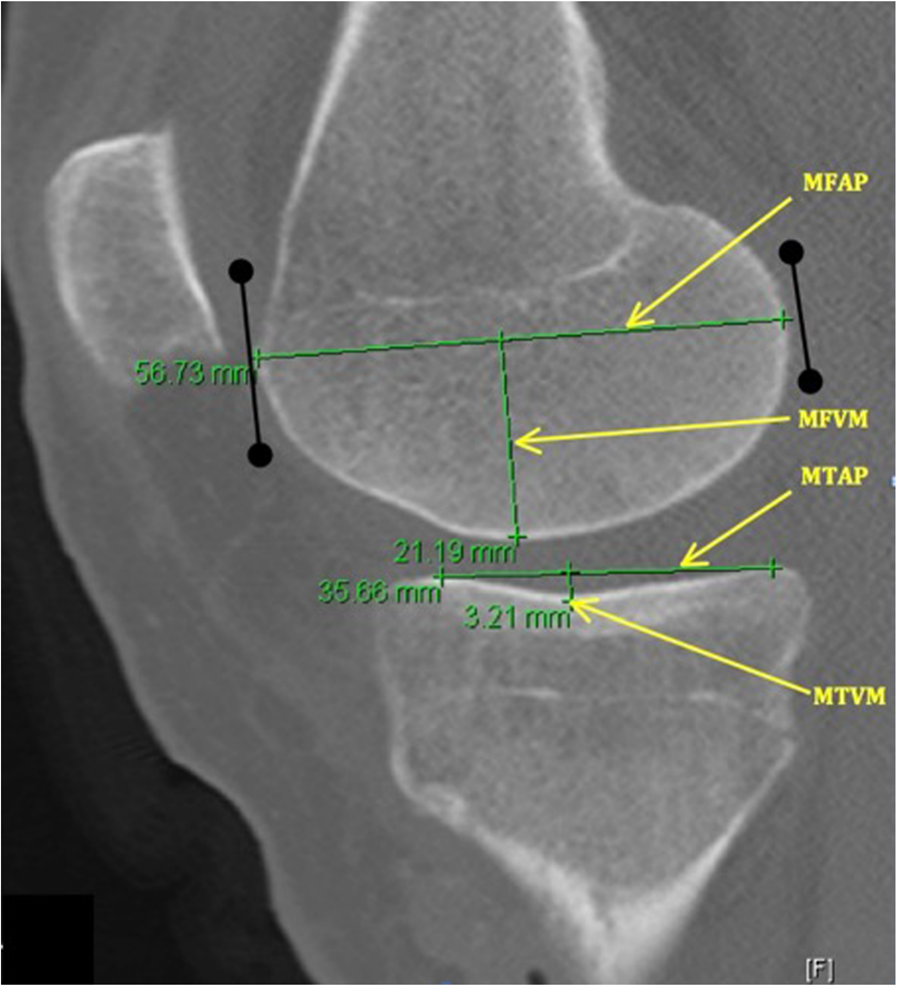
Measurements on a sagittal CT reconstruction of the medial compartment. MFAP: medial femoral condyle antero-posterior length. MFVM: medial femoral vertical maximum of the curvature. MTAP: medial tibial condyle antero-posterior length. MTVM: medial tibial plateau vertical maximum of the curvature
